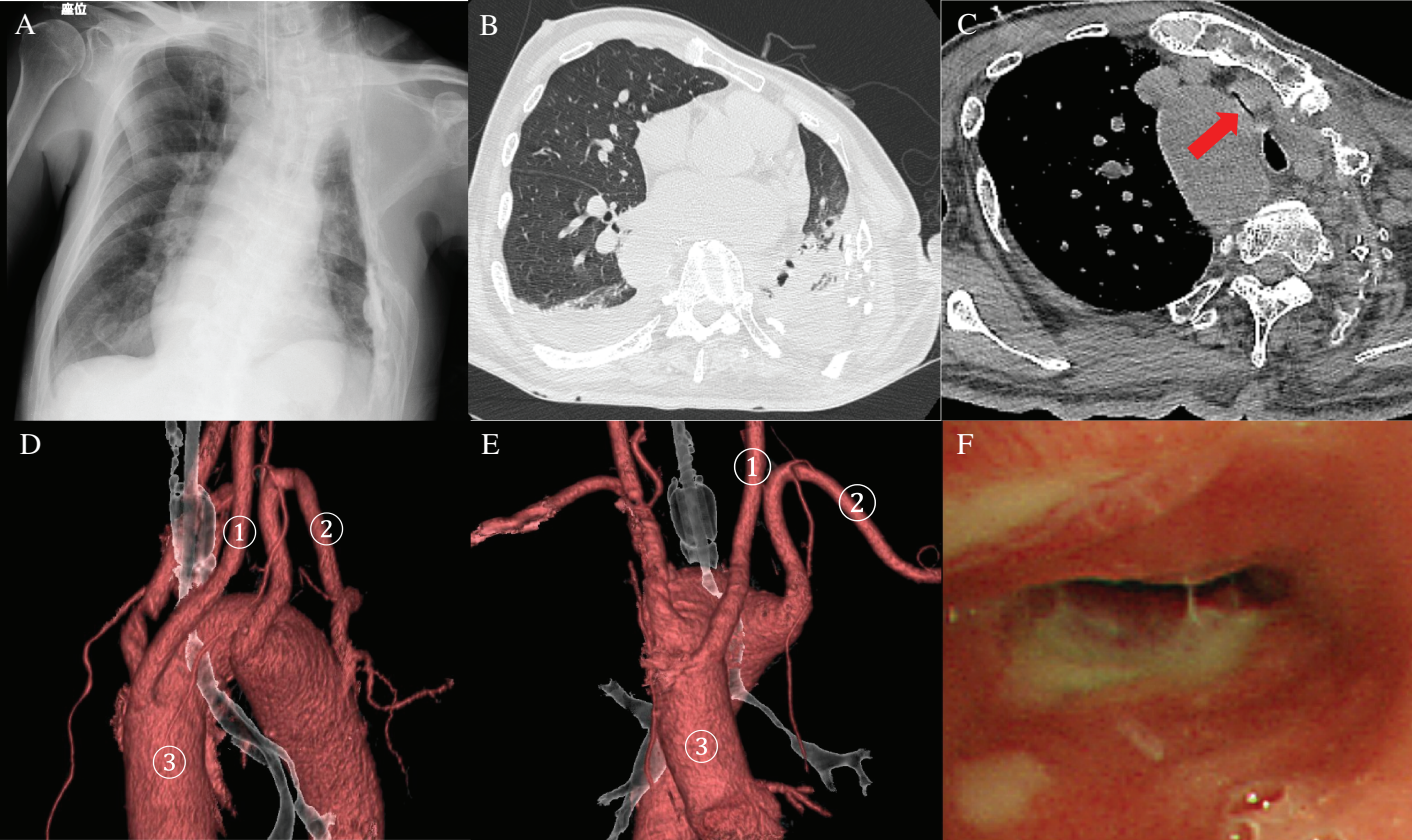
(A) Chest X‐ray showed infiltrative opacities in the lower fields of the left lung. (B) Chest CT (lung window) revealed an infiltrative shadow in the left lower lobe. (C) Chest CT (mediastinal window) showed tracheal compression between the right aortic arch and left common carotid artery with slit‐shaped stenosis (arrow). Using customizable CT image software (DICOM Viewer‐EV Insite R; PSP Corporation, Tokyo, Japan), we estimated a 96.5% stenosis rate with compression of the cross‐sectional area to 11.60 mm^2^ compared to 3 cm^2^ in the upper tracheal segment. (D, E) Chest CT with 3‐dimensional image reconstruction revealed that the trachea was compressed between the right aortic arch(③), and the left common carotid artery with an aberrant origin(①). ②: the left subclavian artery. (F) Bronchoscopic findings revealed redness and partial ulceration of the bronchial epithelium in the area of constriction.
